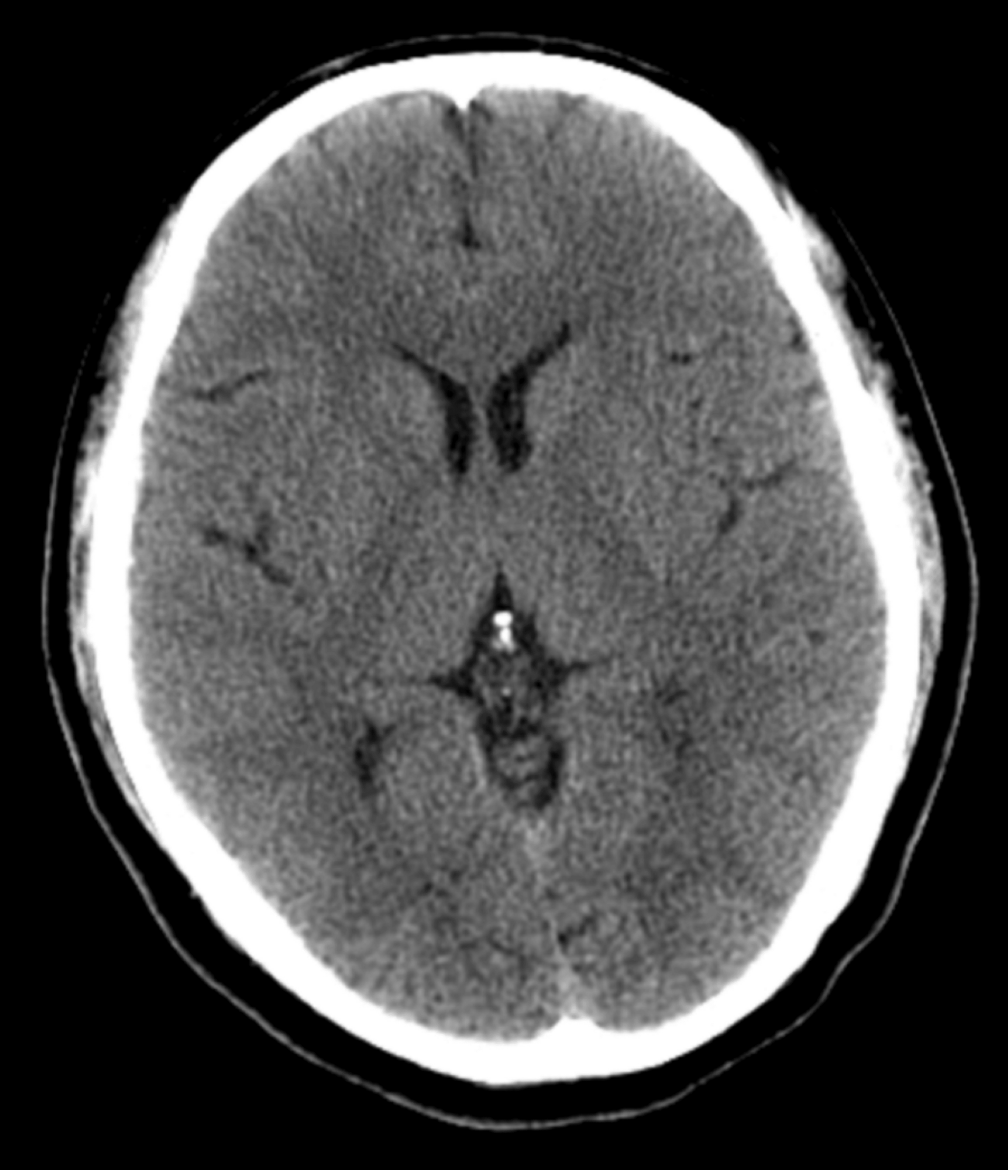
Non-contrast cranial CT scan revealed no acute lesions following the cardiorespiratory arrest CT: computed tomography
